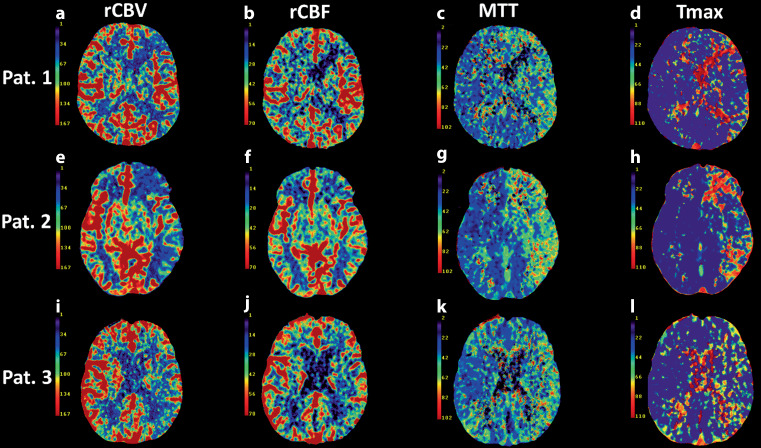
Perfusion parameters of patients with a dissection, an embolic and a macroangiopathic occlusion of the internal carotid artery (ICA). Computed tomography perfusion parameters including *rCBF* (a, e, i), *rCBV* (b, f, j), *MTT* (c, g, k) and *Tmax* (d, h, l) of a patient with a dissection (*Pat.* *1*, a–d), an embolic (*Pat.* *2*, e–h) and a patient with a macroangiopathic occlusion (*Pat.* *3*, i–l) of the left ICA each, the patient with an embolic ICA occlusion had the proportionally most pronounced left hemispheric perfusion deficit. *Pat.* patient, *rCBV* relative cerebral blood volume, *rCBF* relative cerebral blood flow, *MTT* mean transit time, *Tmax* time to maximum
